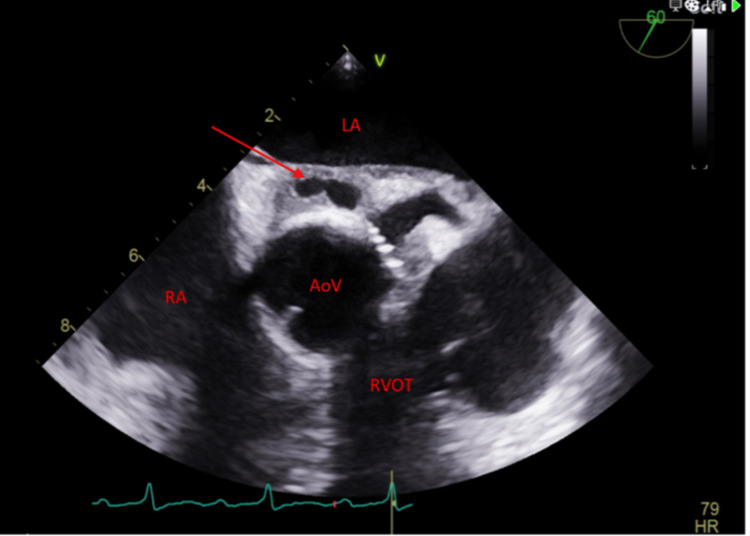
Transesophageal echocardiogram with midesophageal aortic valve view depicting aortic valve with aortic root abscess marked by a red arrow, with an echo-lucent channel centrally. Profound first-degree heart block is also observed. AoV, aortic valve; Ao, aorta; RA, right atrium; LA, left atrium; RV, right ventricle; LV, left ventricle
